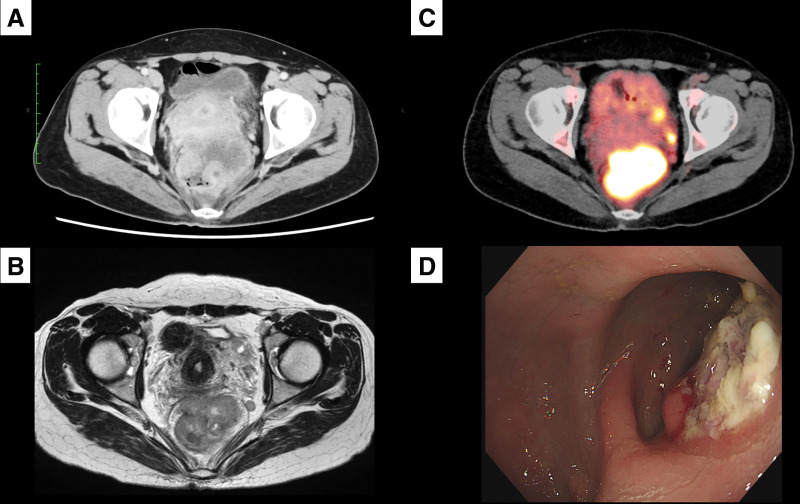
Preoperative multimodal imaging studies. (A) Contrast-enhanced abdominal CT demonstrating a highly invasive, bulky tumor occupying more than half of the pelvic cavity and involving both the rectum and the uterus. (B) Pelvic MRI showing circumferential rectal wall thickening with heterogeneous enhancement, suggestive of invasion into the uterovaginal junction. (C) Fluorine-18 fluorodeoxyglucose-PET revealing marked tracer uptake in the rectal tumor as well as in the bilateral lateral pelvic lymph nodes. (D) Repeat colonoscopy identifying the lower tumor margin approximately 6 cm from the anal verge. Extramural compression was again observed, accompanied by partial mucosal ulceration with a villous tumor-like appearance.
